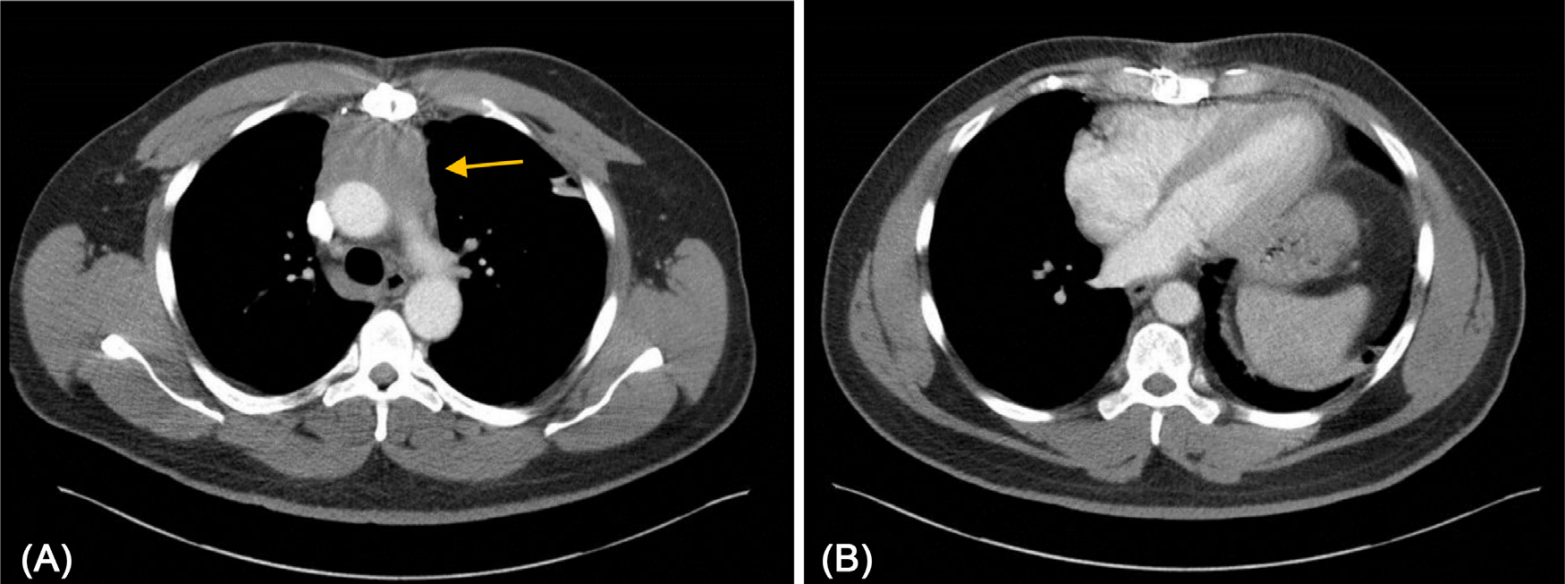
Axial CT imaging from 2016 showing (A) a homogeneous lobulated mass (yellow arrow) at the anterior mediastinum measuring 7.6 × 5.6 cm confirmed to be a thymoma. (B) normal presternal region prior to radiation therapy.
